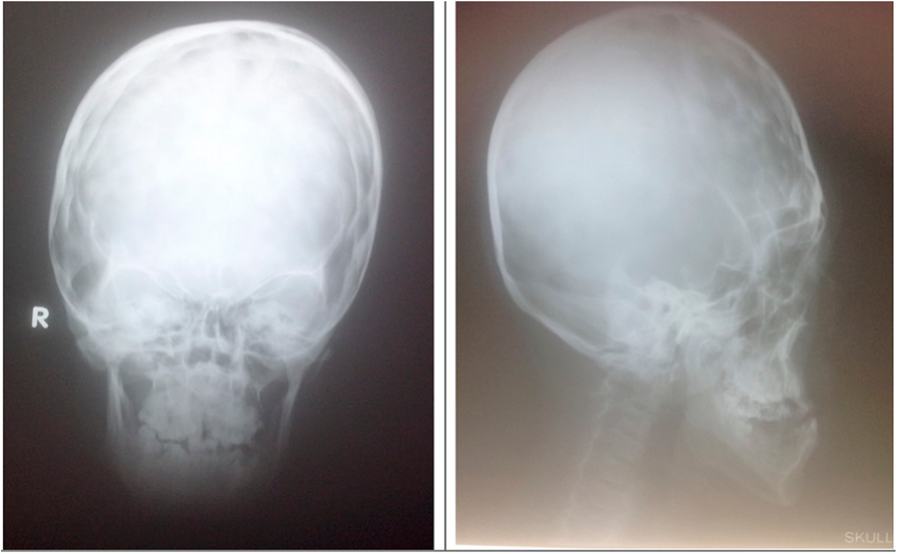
Anterior and lateral pictorial and X-ray views of the face
